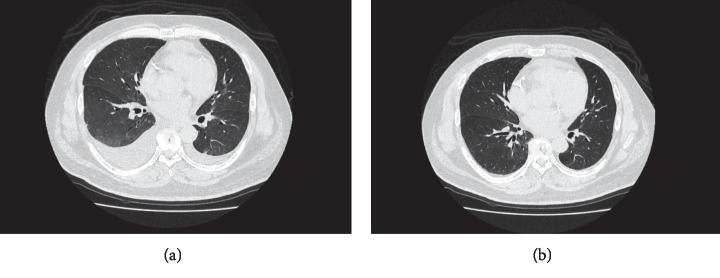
(a) Chest CT four months after brodalumab initiation, which showed bilateral ground-glass opacities and pleural effusions. (b) Seven months after brodalumab initiation, bilateral ground-glass opacities and pleural effusions improved.
